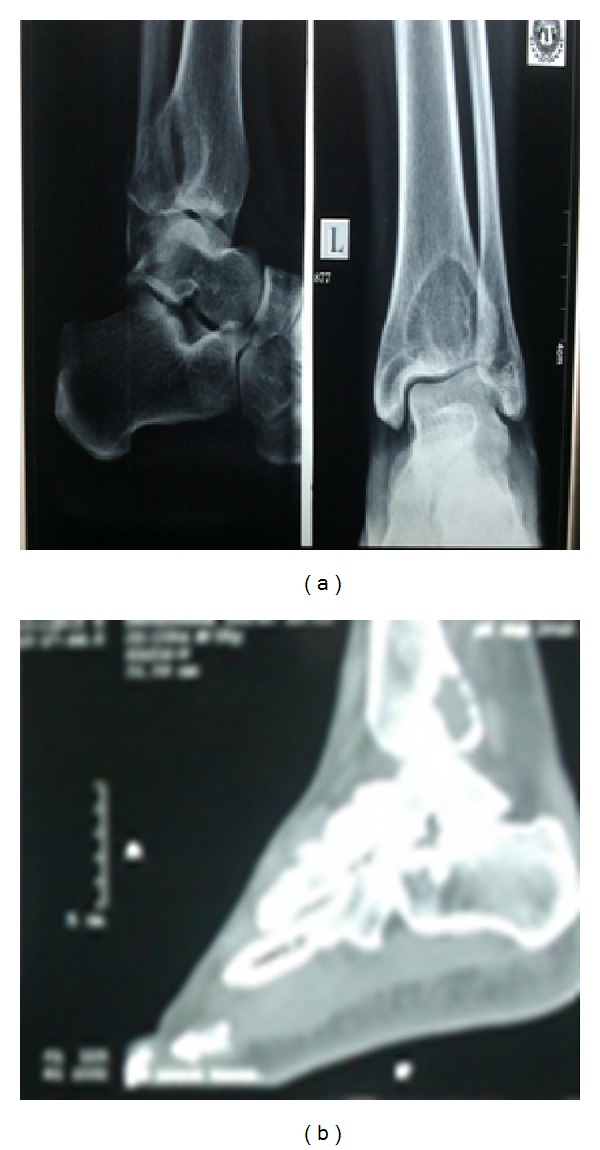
Preop X-ray and CT scan showing osteolytic lesion of distal tibia.
